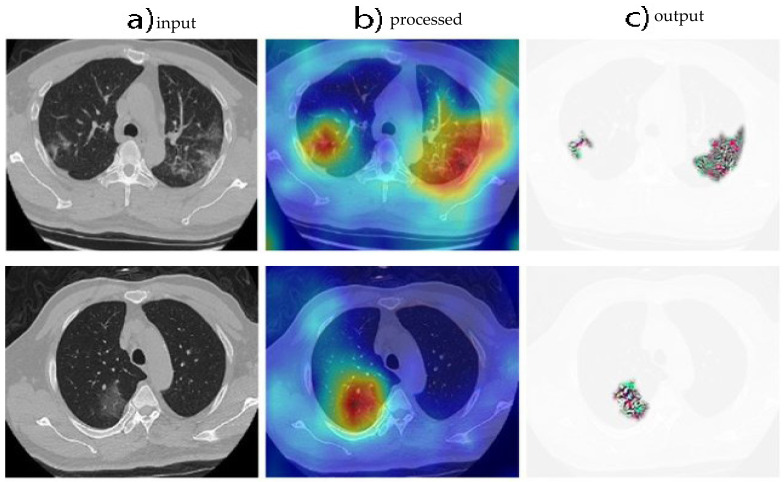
Grad-CAM visualization of model predictions on CT images.
